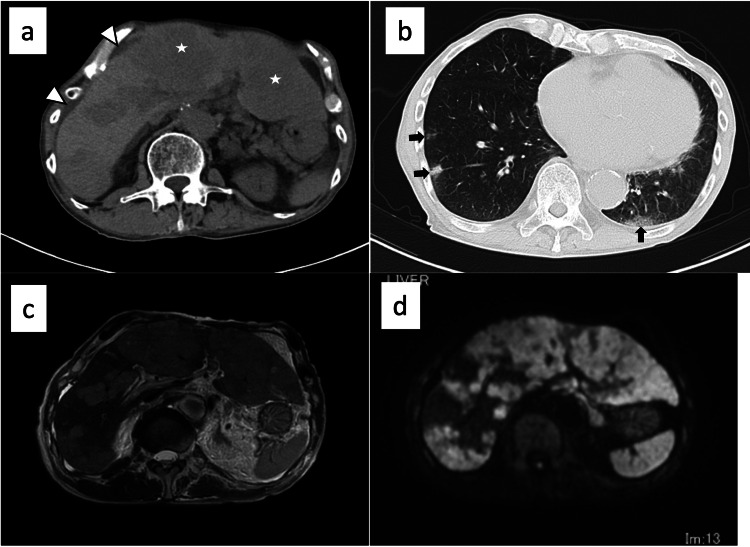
Imaging findings. Non-contrast computed tomography (CT) image (a, b). (a) Abdominal CT scan revealed low-density lesions in the liver (stars), mainly left but also right lobe, and a small amount of ascites (arrowheads). These findings suggested malignant tumors, including metastatic liver tumors. (b) Chest CT scan showed multiple ground-glass opacity and infiltrate shadows in the bilateral peripheral lungs (black arrows), suggesting metastatic lung tumors.
